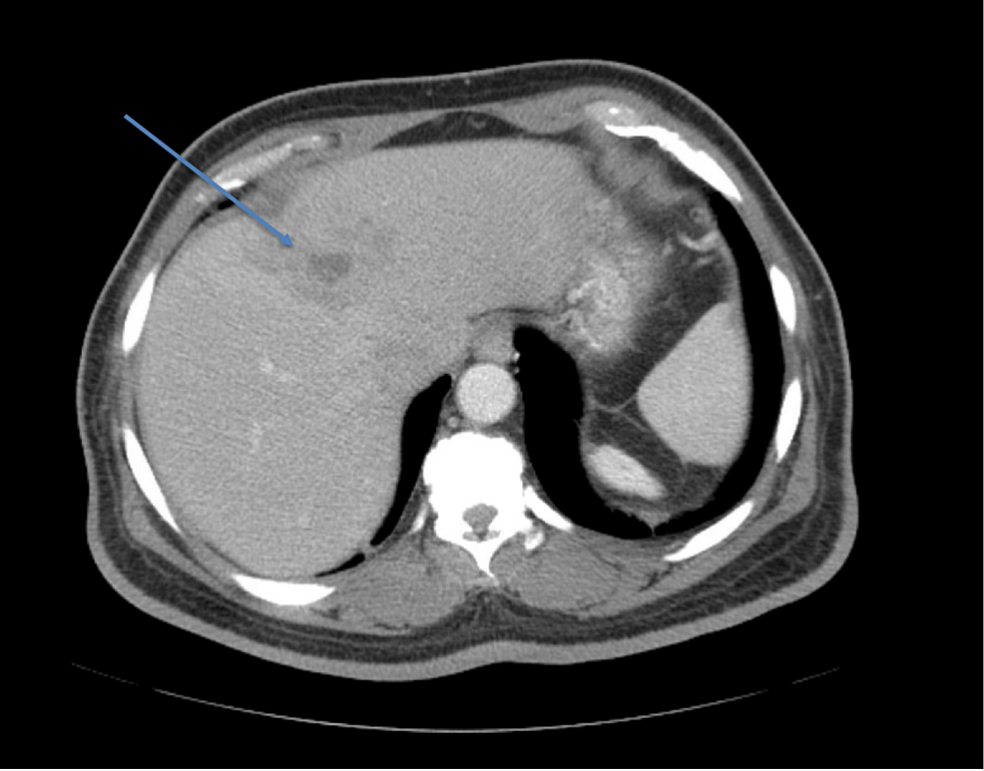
CT abdomen with intravenous (IV) contrast. Heterogeneous segment 4A with a near-complete resolution of the previous collection, and the residual discrete dominant component measuring approximately 2.1 cm x 1.6 cm x 2.0 cm.
